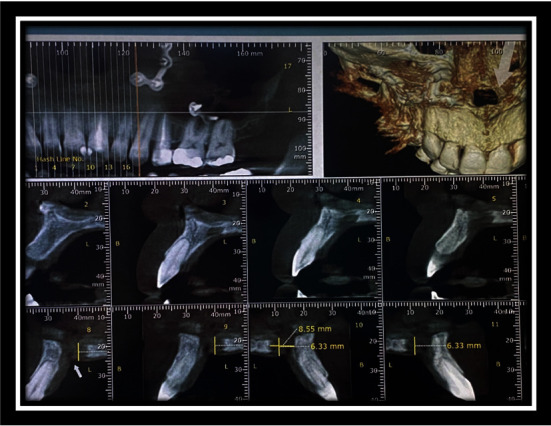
CBCT after fistula formation.
